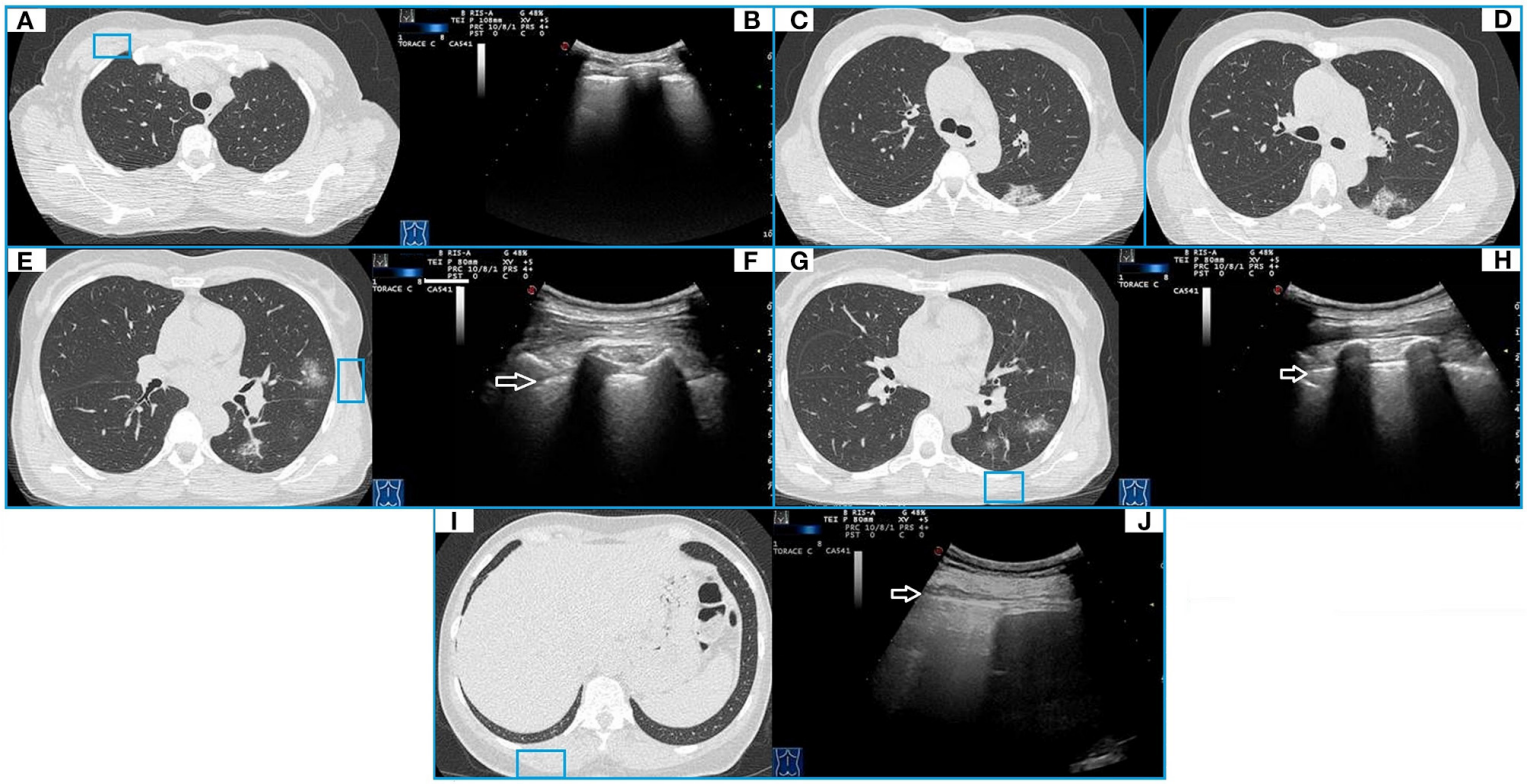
CT and TUS findings in mild COVID-19 pneumonia. A 57-year-old female patient presenting in ED with a 1 week fever, dyspnea, cough, and fatigue. The RT-PCR assay on nasopharyngeal swab confirmed the suspect for COVID-19 pneumonia. In (A), axial CT scan passing through the upper lobes shows a peripheral focal ground glass opacity located in the anterior parenchyma of right upper lobe and not adhering to the pleural surface. In (B), ultrasound scan with a convex probe (6 MHz) and thoracic setting in the upper region of the chest [blue box in (A)] shows a normal hyperechoic pleural line (white arrow) without B-lines. Axial CT scans passing through the tracheal carina [in (C)] and the middle lung regions [in (D)] show mixed areas of ground glass/consolidation with subpleural distribution in the left lower lobe. These lesions are located in the retroscapular area, resulting in being not visible on ultrasound scans. Axial CT scans passing through the middle lung regions [in (E)] and the lower lobes [in (G)] show rounded focal ground glass opacities with central distribution in the lingula and the left lower lobe. In (F,H), ultrasound scans with a convex probe (6 MHz) and thoracic setting, corresponding to the blue boxes in the respective CT scans, show a normal hyperechoic pleural line without B-lines (white arrow). In (I), axial CT scan passing through the basal lung regions shows no significant lesions. In (J), ultrasound scan with a convex probe (6 MHz) and thoracic setting, corresponding to the blue box in the respective CT scan, shows a normal hyperechoic pleural line (white arrow) near the correspondent right posterior costofrenic sinus.
